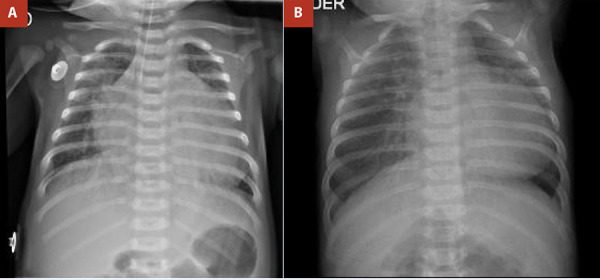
Chest radiograph. A) Case 01. B) Case 02. Dilatation of the left cardiac chambers with bilateral pulmonary overcirculation.
